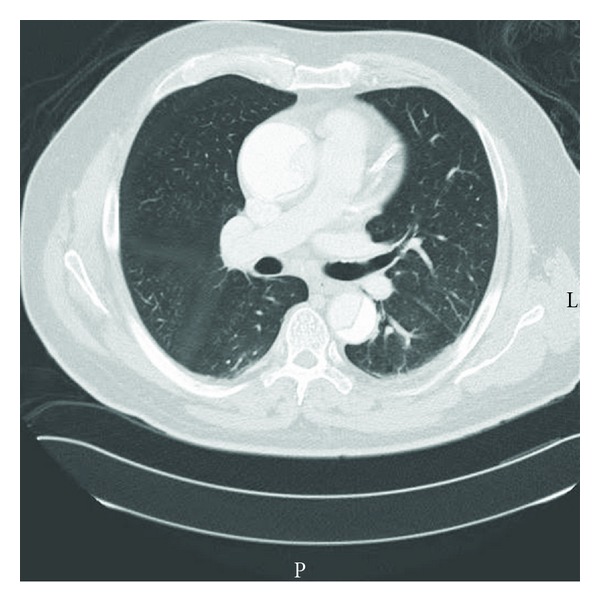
Axial computed tomography scan. Image shows thoracic aortic dissection (De Bakey/Standford type I/A).
